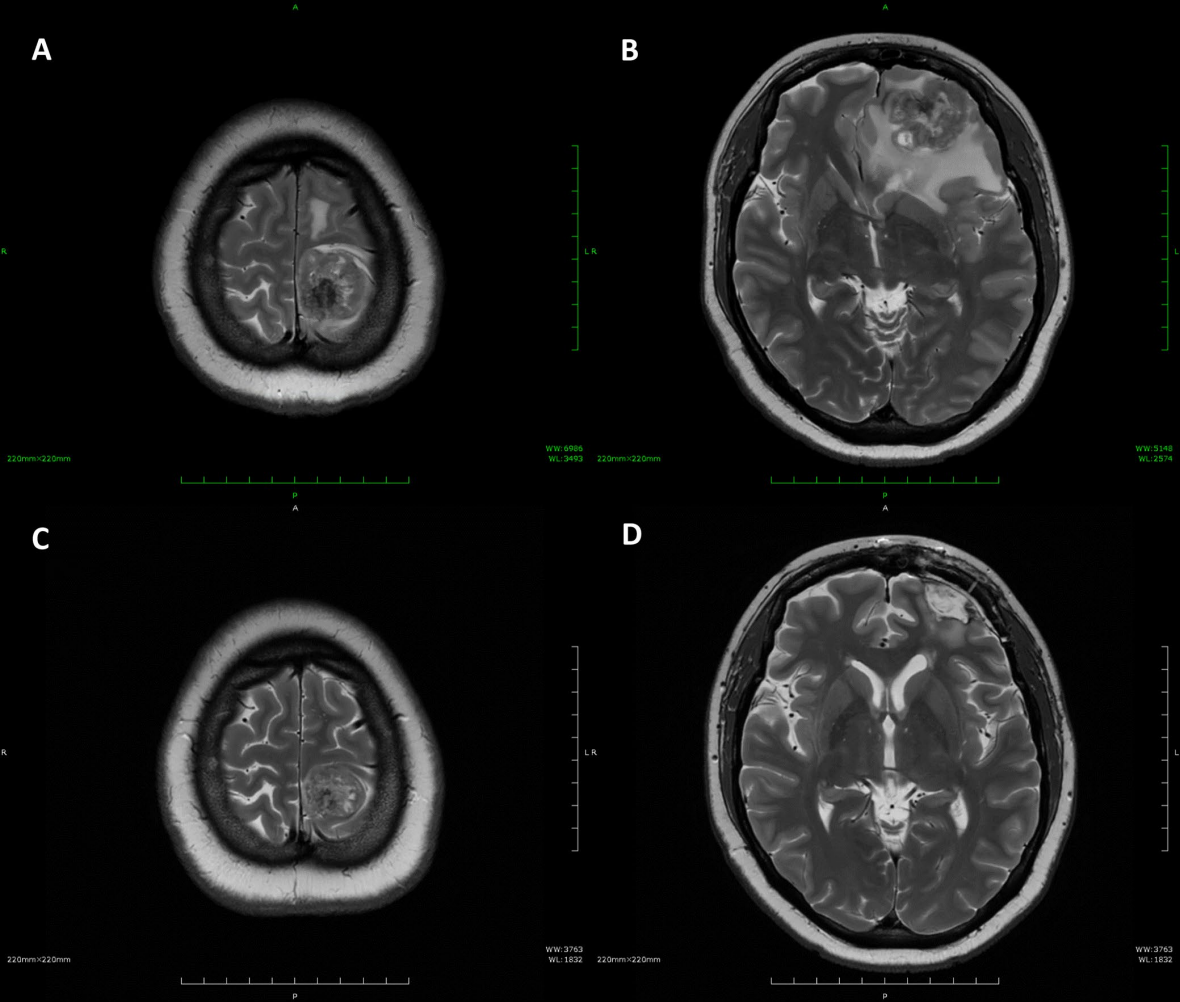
Magnetic resonance imaging of the brain. (A) T2‐weighted images revealed a substantial mass in the parietal lobe. (B) T2‐weighted images revealed a mass with midline shift in the frontal lobe. (C) T2‐weighted images showed shrinkage of the parietal lobe mass after irradiation and lenvatinib plus pembrolizumab treatment. (D) T2‐weighted images showed shrinkage of the frontal lobe mass after surgery, irradiation and lenvatinib plus pembrolizumab treatment.
